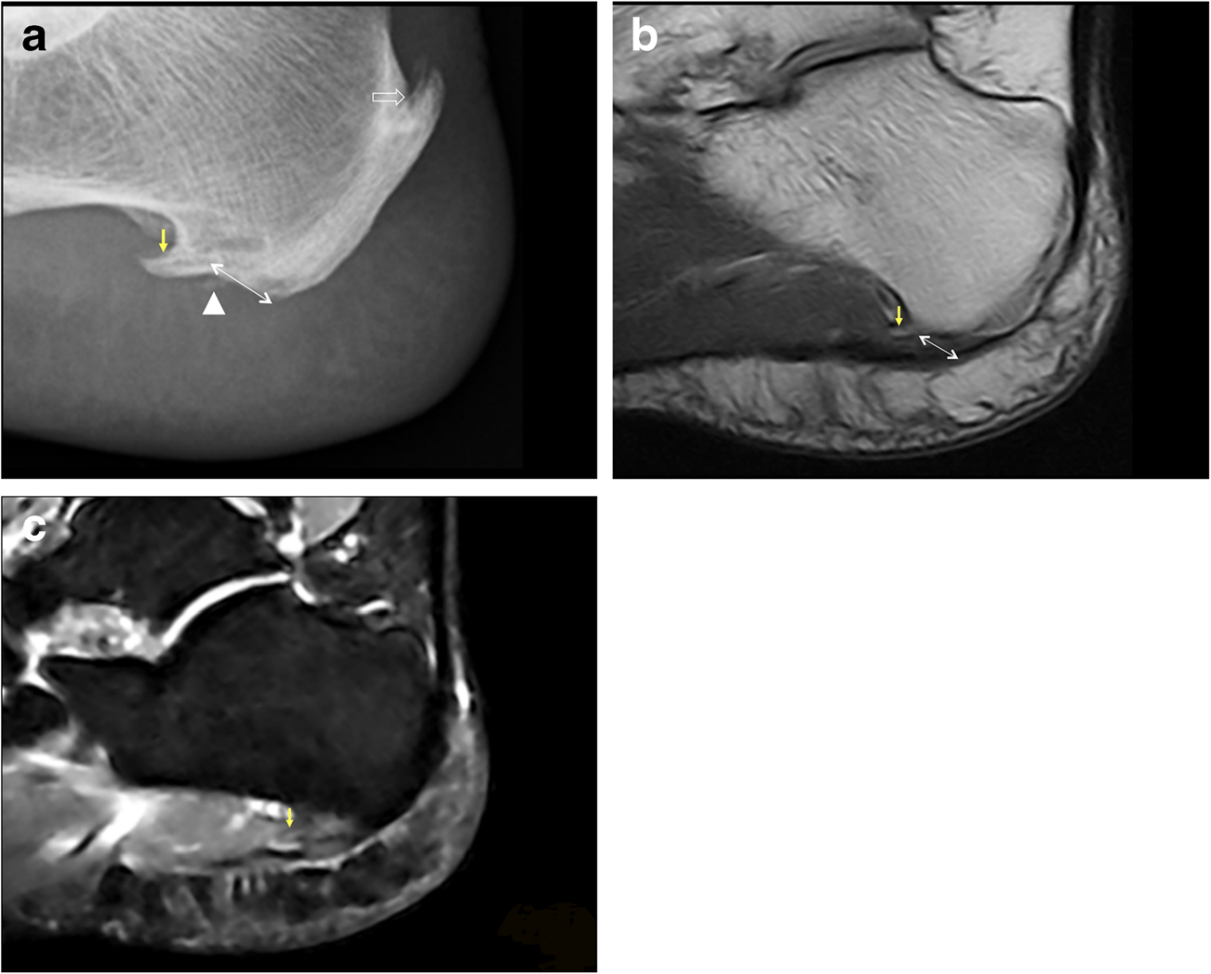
Plantar fasciitis. Lateral plain radiograph shows PF thickening (*double-head arrow*) and fine calcifications at the calcaneal insertion of the PF (*arrowhead*); a plantar calcaneal spur at the origin of intrinsic muscles of the foot (*arrow*) and calcific enthesopathy of the Achilles tendon (*open arrow*) are also evident (a). MRI confirms the presence of a calcaneal spur (*arrow*) and PF thickening at its calcaneal attachment (*double-head arrow*) (b). Bone marrow oedema in the calcaneal spur (*arrow*) is demonstrated on the fluid-sensitive image (c)
